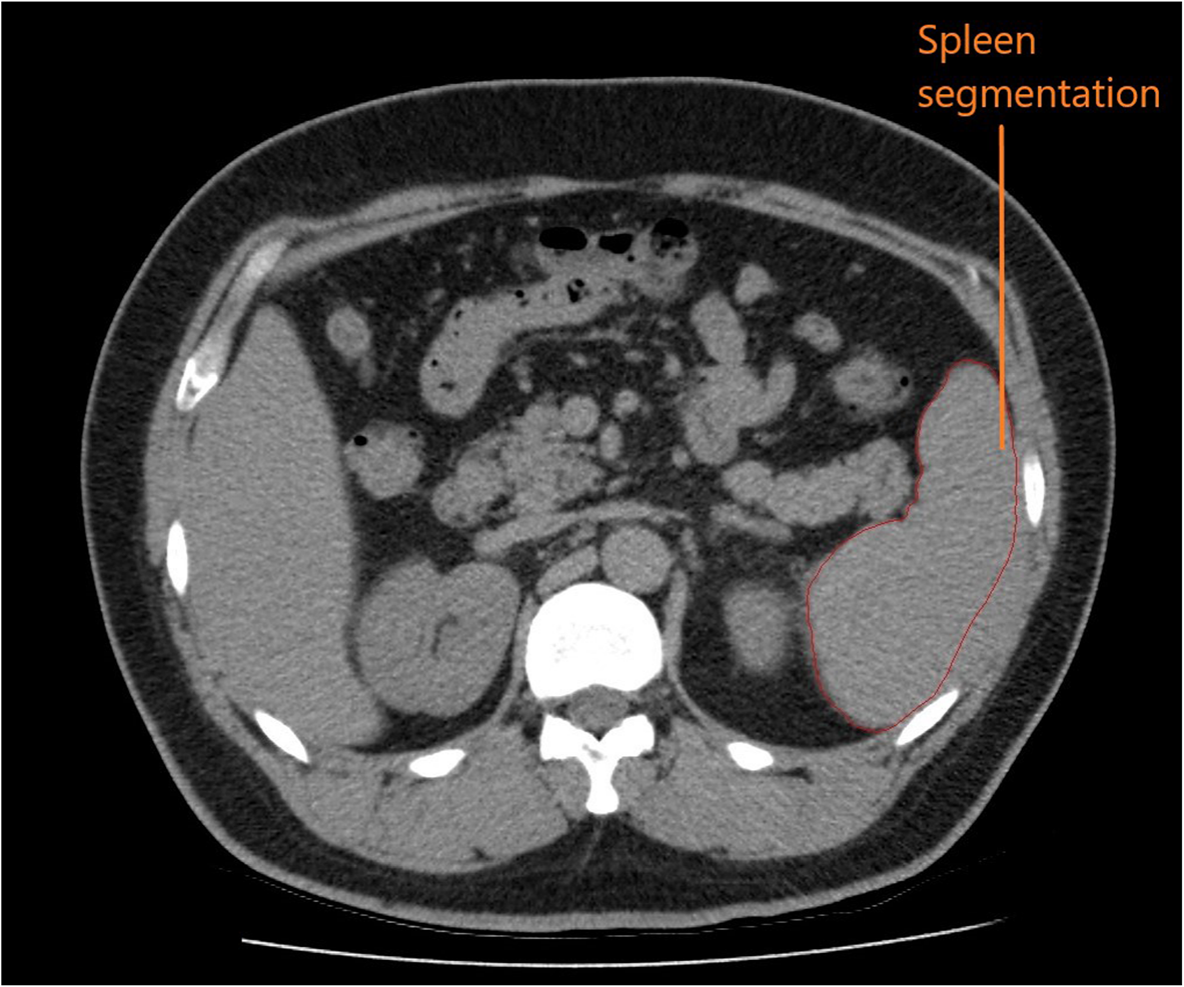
Manual segmentation was performed on the spleen and other organs using the transaxial images from the abdominal CT scan
